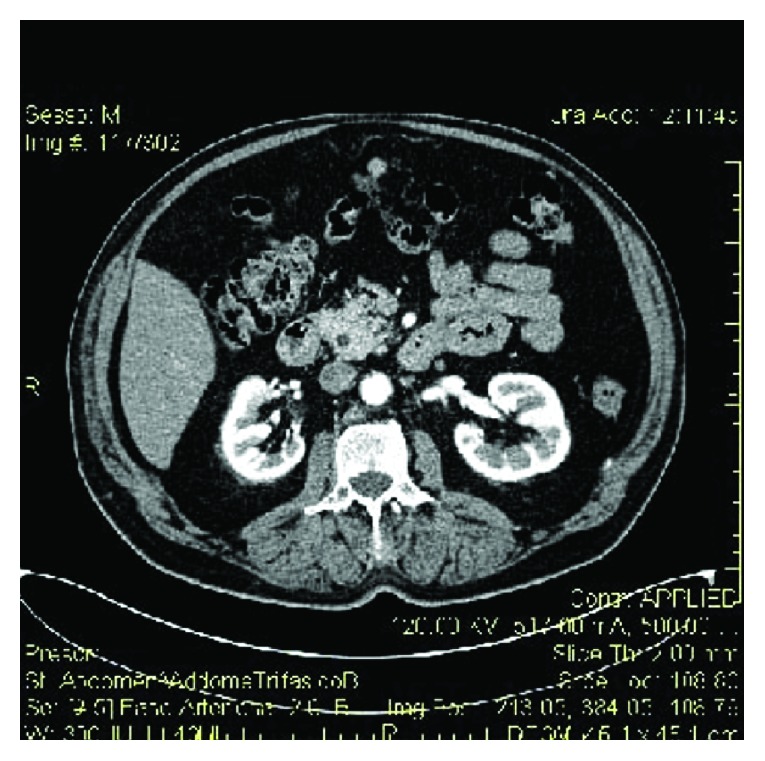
Follow-up CT arterial phase.
